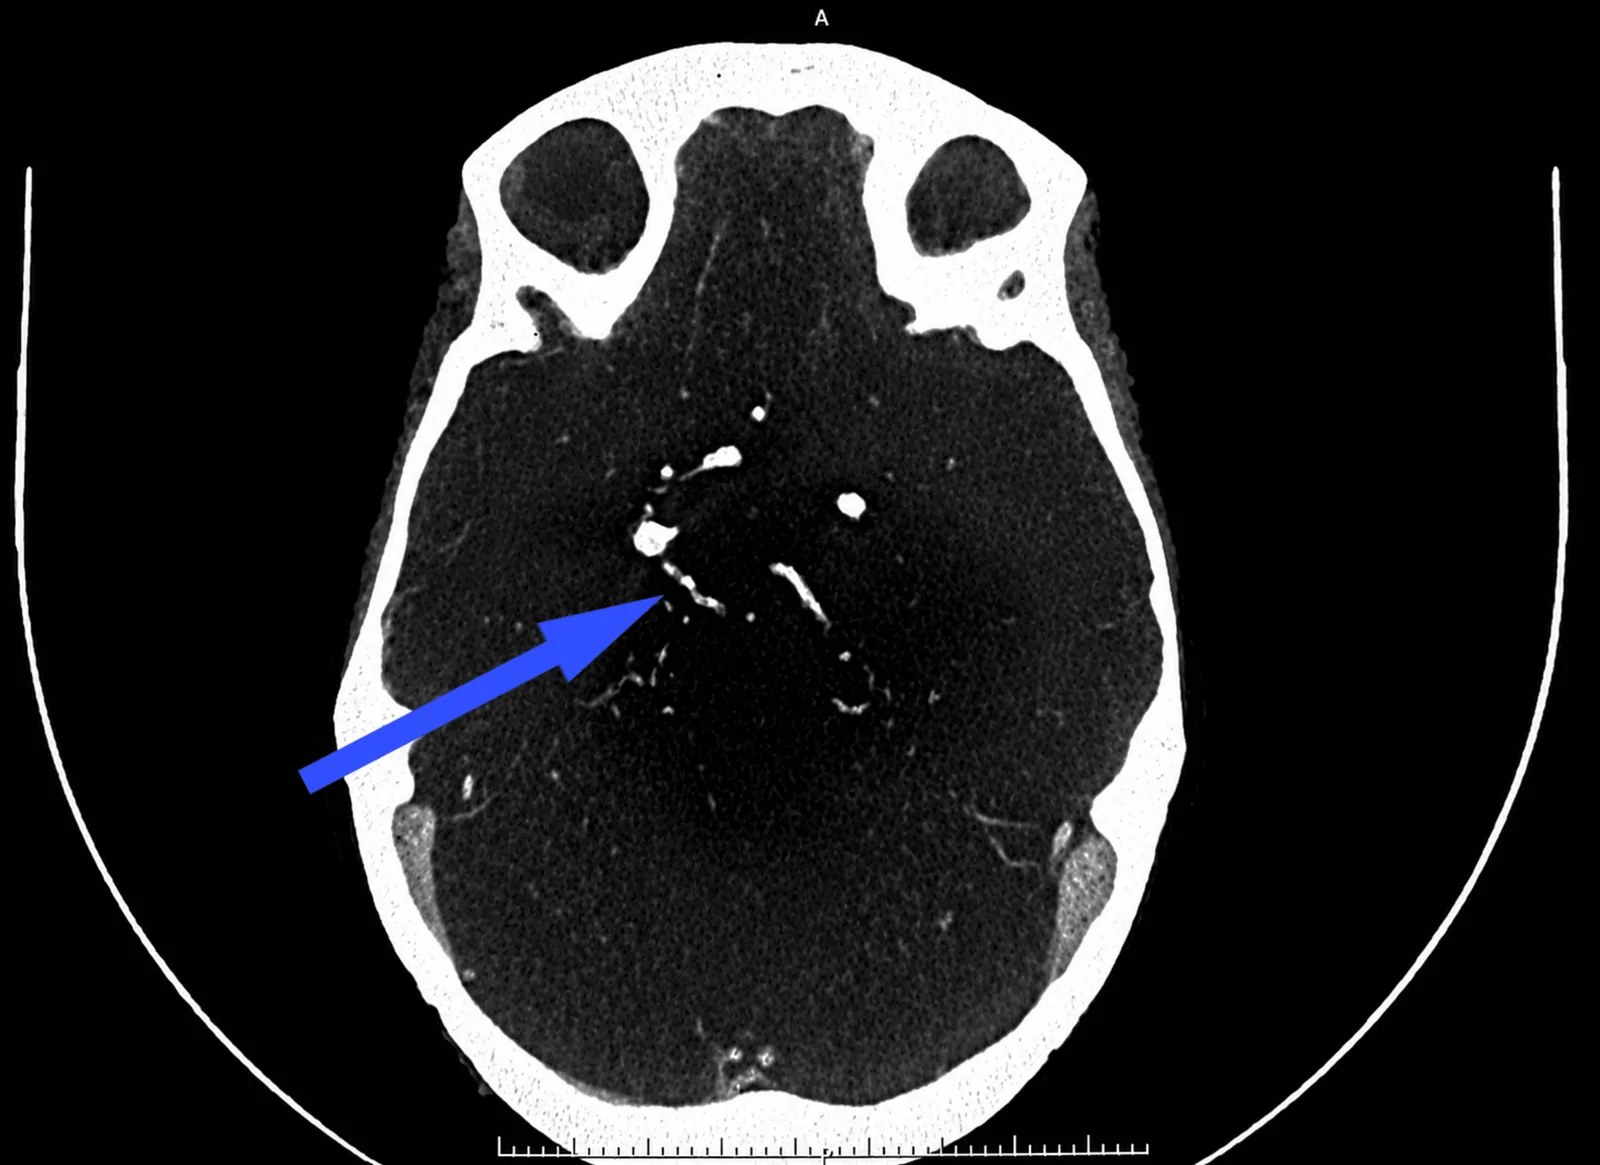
Carotid and cerebral computed tomographic angiography The P1 segment of the right posterior cerebral artery is hypoplastic, and the P2 segment is seen as a continuation of the right posterior communicating artery ( blue arrow), suggestive of fetal origin of the right posterior cerebral artery.
